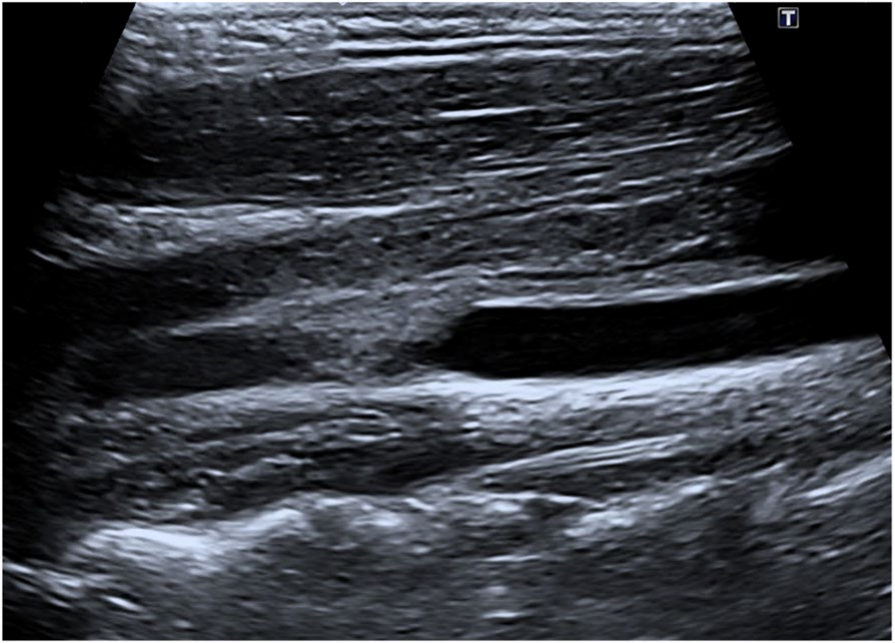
Ultrasound-jugular venous system: partial filling by echogenic material, compatible with thrombosis
